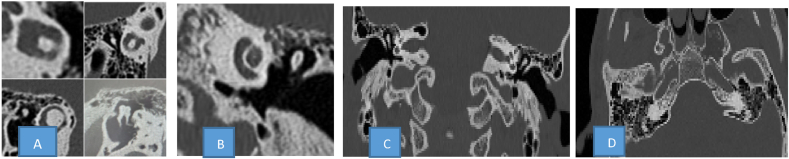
CT-scan of petrous bone was normal: labyrinths, semicirculars canals, the ossicles of middle ear, tympanic cavity, inner ear structures are normal. Hyper-pneumatazetion of mastoid cells and lesion of left sphenoid bone. Presence of a hypodense lesion in the left sphenoid bone.
